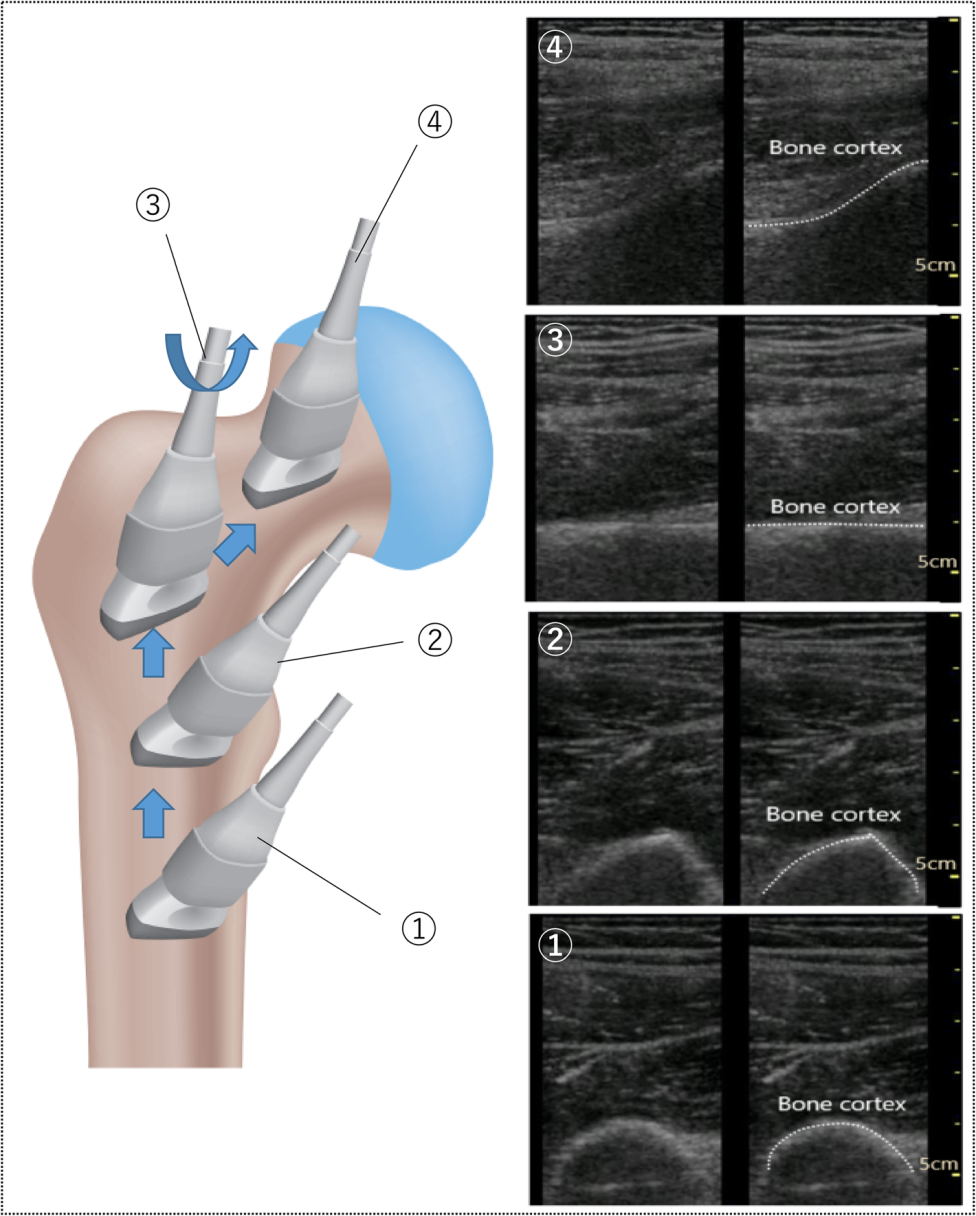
Our stylized handling of the ultrasound probe to detect hip joint fracture. The ultrasound probe was moved in the order from ① to ④. This figure was drawn up by TK (author)
